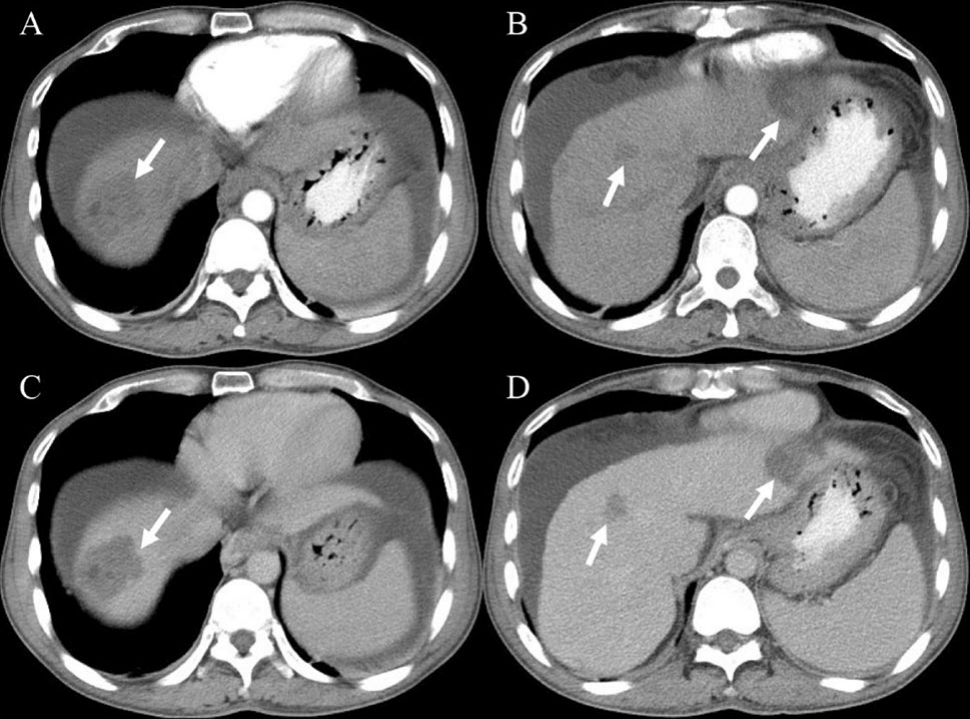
A case of multiple hepatic sclerosed hemangiomas was misdiagnosed as a possible malignant disease (male, 50-years-old). Axial CT in the arterial phase and delayed phase demonstrated multiple irregular heterogeneous hypodense lesions in the liver with no obvious enhancement (A–D) (arrows)
